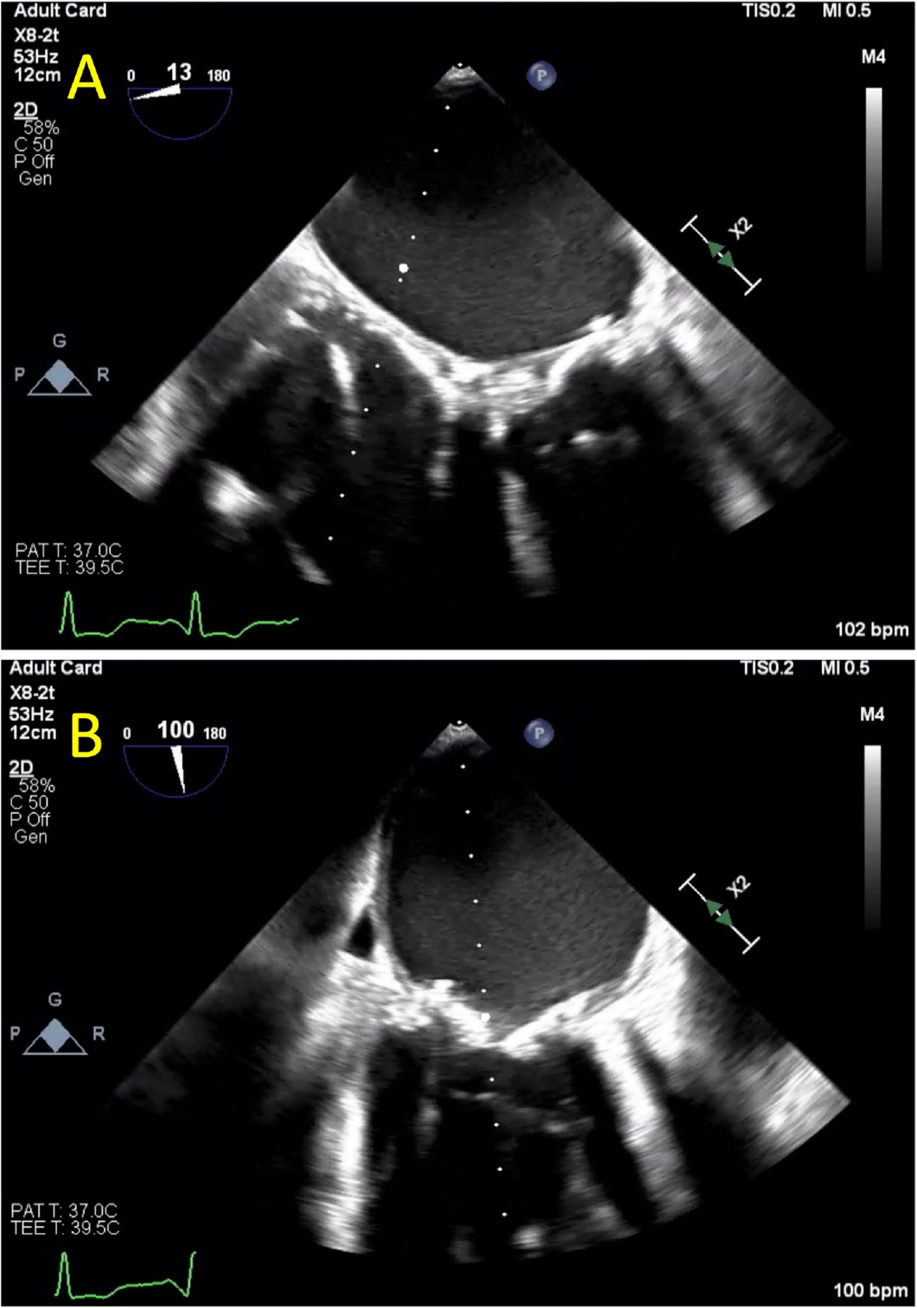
Echocardiographic images of bioprosthetic mitral valve in mid-esophageal 4 chamber and long axis view following left atrial thrombus resolution. Transesophageal echocardiogram (TEE) performed approximately 4 weeks after left atrial thrombus was identified showing resolution of thrombus in end-diastole in the (A) mid esophageal 4 chamber view at 13 degrees and (B) long axis view at 100 degrees. Severe leaflet restriction is noted, and the mean gradient was measured at 26 mmHg although at a heart rate of 100 beats per minute
